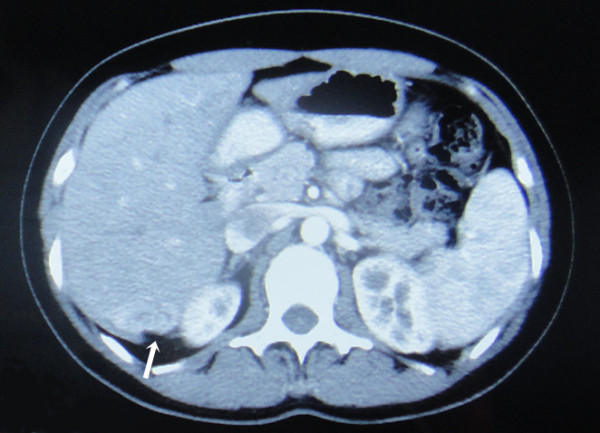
Contrast-enhanced CT showed a heterogeneously enhanced mass (arrow)
